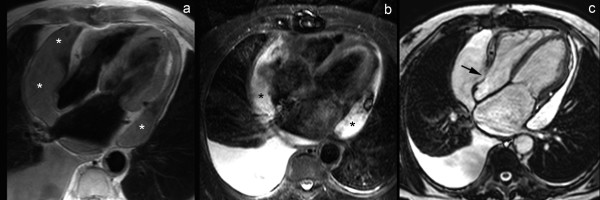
Moderate pericardial effusion. T1-weighted spin-echo CMR. (a), T2-weighted short-tau inversion-recovery spin-echo CMR (b), and cine CMR (c) in axial image plane. The pericardial effusion (arrowheads), having an inhomogeneous spread, is most pronounced over the right atrium and left ventricle. Systolic collapse of the right atrial wall during systole (arrow)(c). The fluid signal intensity, especially on T2-weighted short-tau inversion-recovery spin-echo CMR, has an inhomogeneous appearance (b). Moderate right-sighted pleural effusion. The collapse of right atrial and right ventricular wall during the cardiac cycle can be well appreciated on cine CMR.
